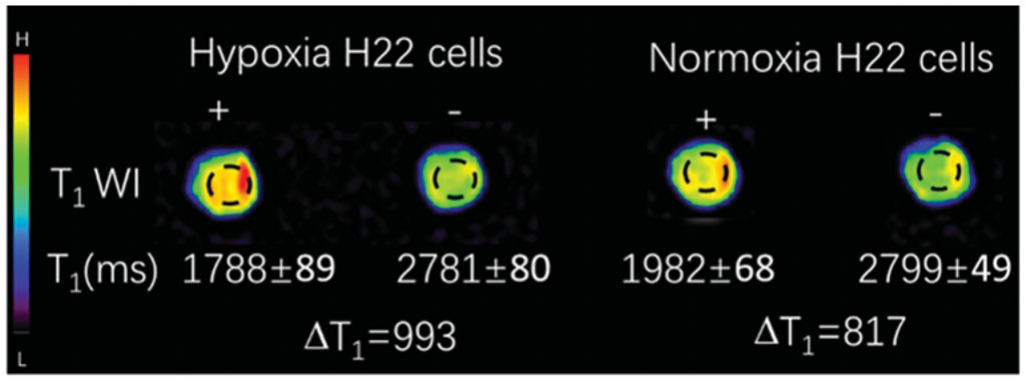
T1-weighted H22 cellular MRI with (+) and without (–) L-EGCG-Mn NPs. The T1 value was obtained from the T1 mapping images. ΔT1 was calculated by subtracting the T1 value for 1 mM Mn from the T1 value for 0 mM Mn.
